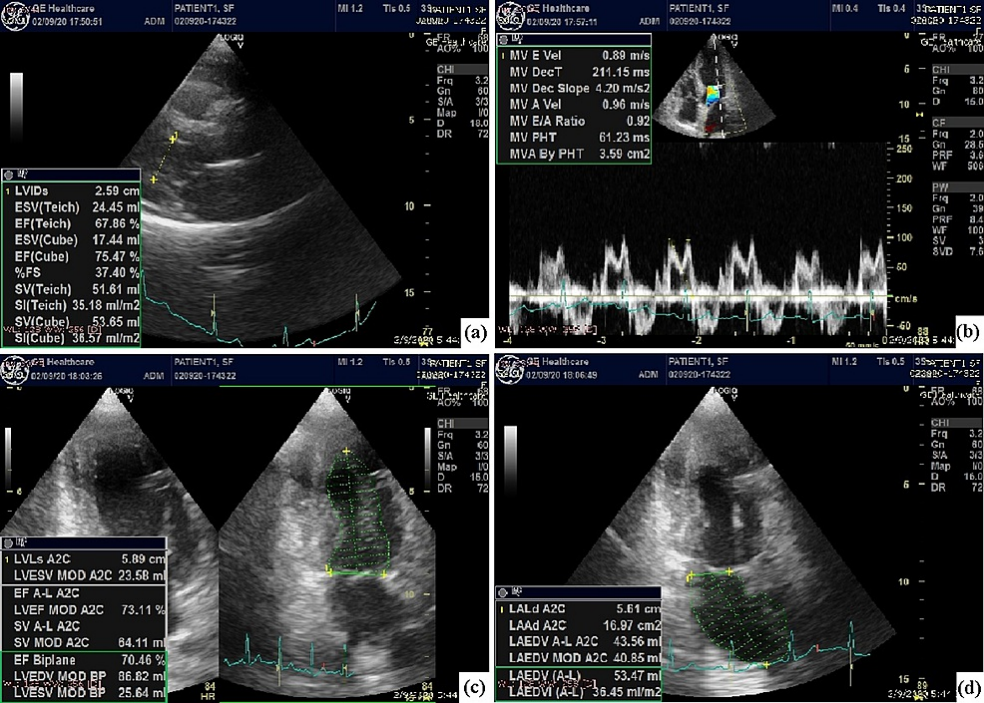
Echocardiographic evaluation of the patient. (a) Parasternal long axis. Normal dimensions of the left atrial and ventricular chambers; (b) transmitral E to A ratio (E≈A);  (c and d)  apical 4 and 2 chambers view. The normal systolic function of the left ventricle (Simson method).
